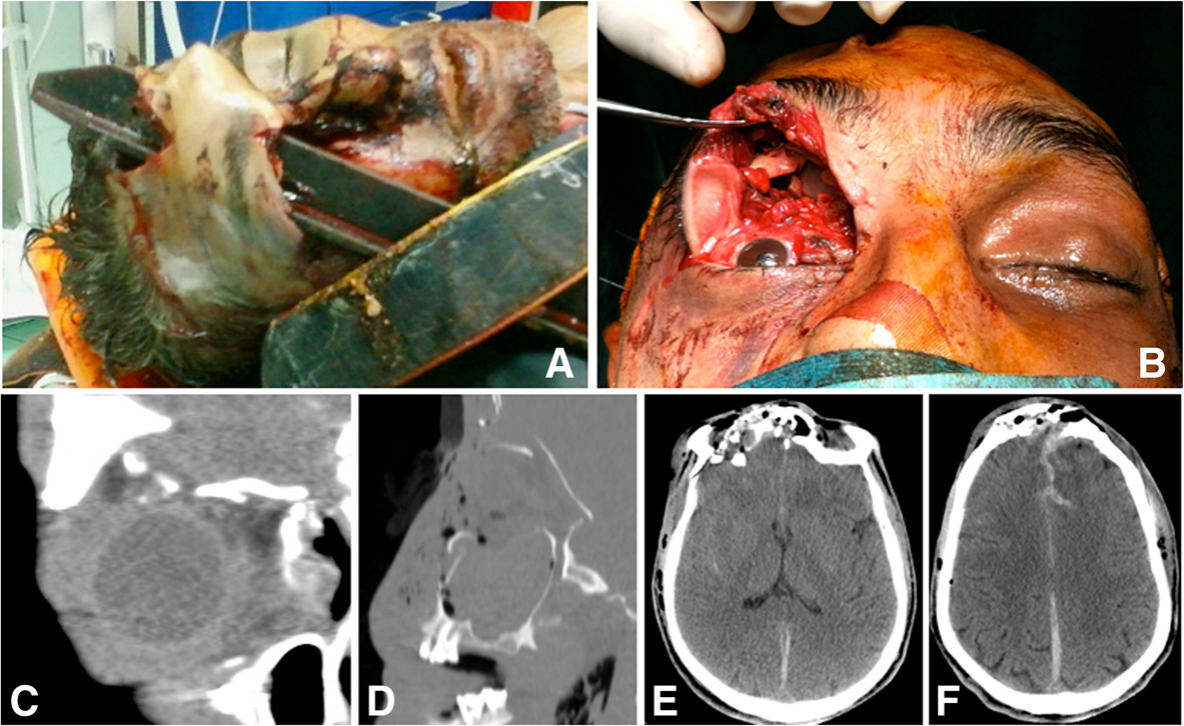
Patient at emergency department. A: Patient at our Emergency Department with the metal rod still embedded into the right orbit and skull. B: Inspection of the right orbit, showing a complete destruction of orbit roof with the ocular globe pushed backwards and downwards. C and D: CT coronal and sagittal scan reconstructions showing the fracture of both orbit floor and roof. E and F: CT axial scans at admittance, demonstrating the fracture of frontal bone, with opening of frontal sinus, and signs of subarachnoid hemorrhage in the inter hemispheric cistern.
